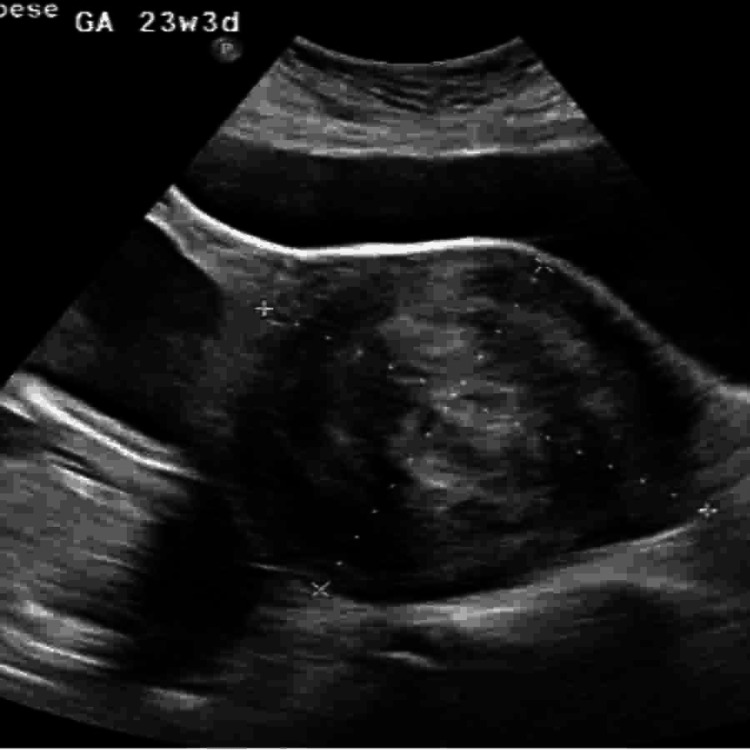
Ultrasound image at 23 weeks and 3 days of gestation showing a large, well-defined hypoechoic uterine fibroid located in the anterior uterine wall. The fibroid measures approximately 11.8×10.6 cm and compresses the adjacent uterine structures, with visible displacement of the fetus.
